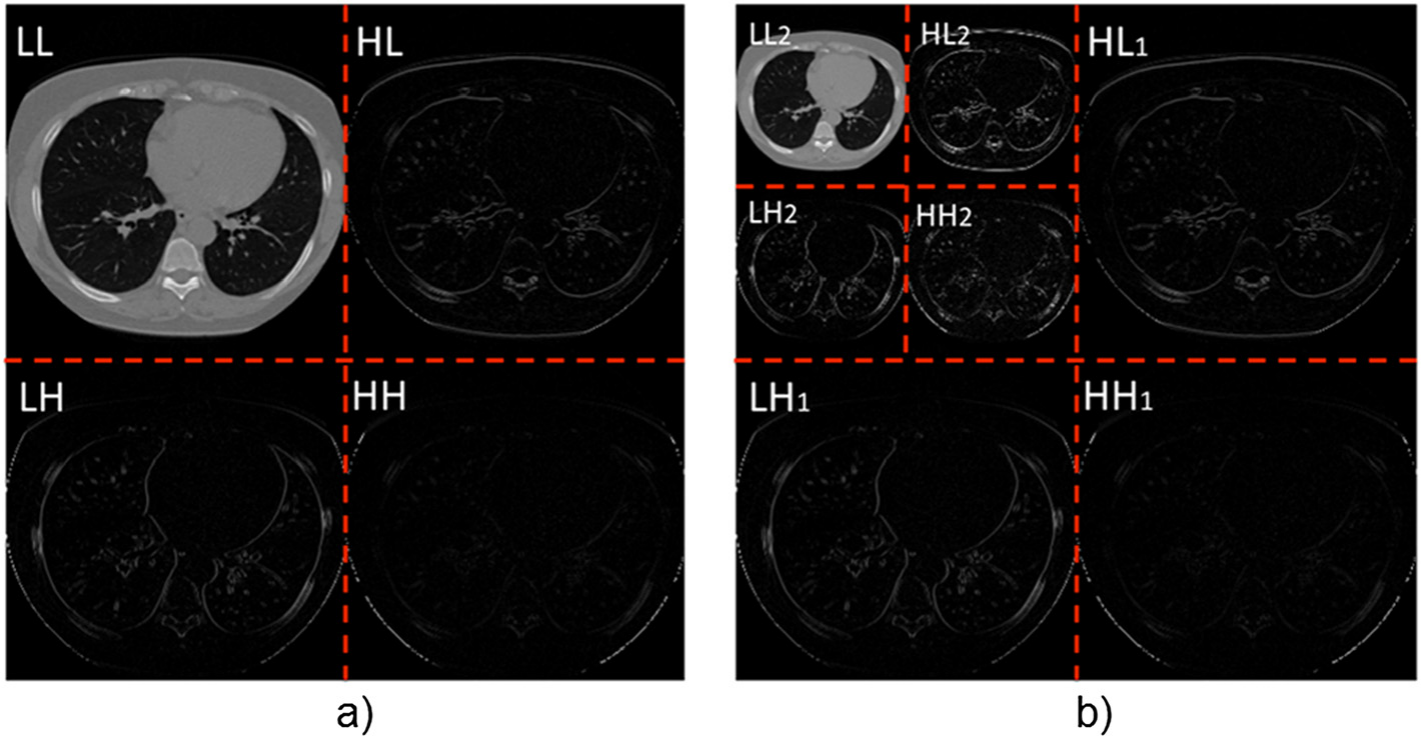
CT image transformed from the original to the wavelet domain with the Daubechies db4 wavelet transform: a) CT image at one decomposition level and b) CT image with two decomposition levels. Notice that the coarse sub-bands captures the information related to the lung nodules. The data from each sub-band defines a nodule candidate, and is used in the stage of feature extraction.
